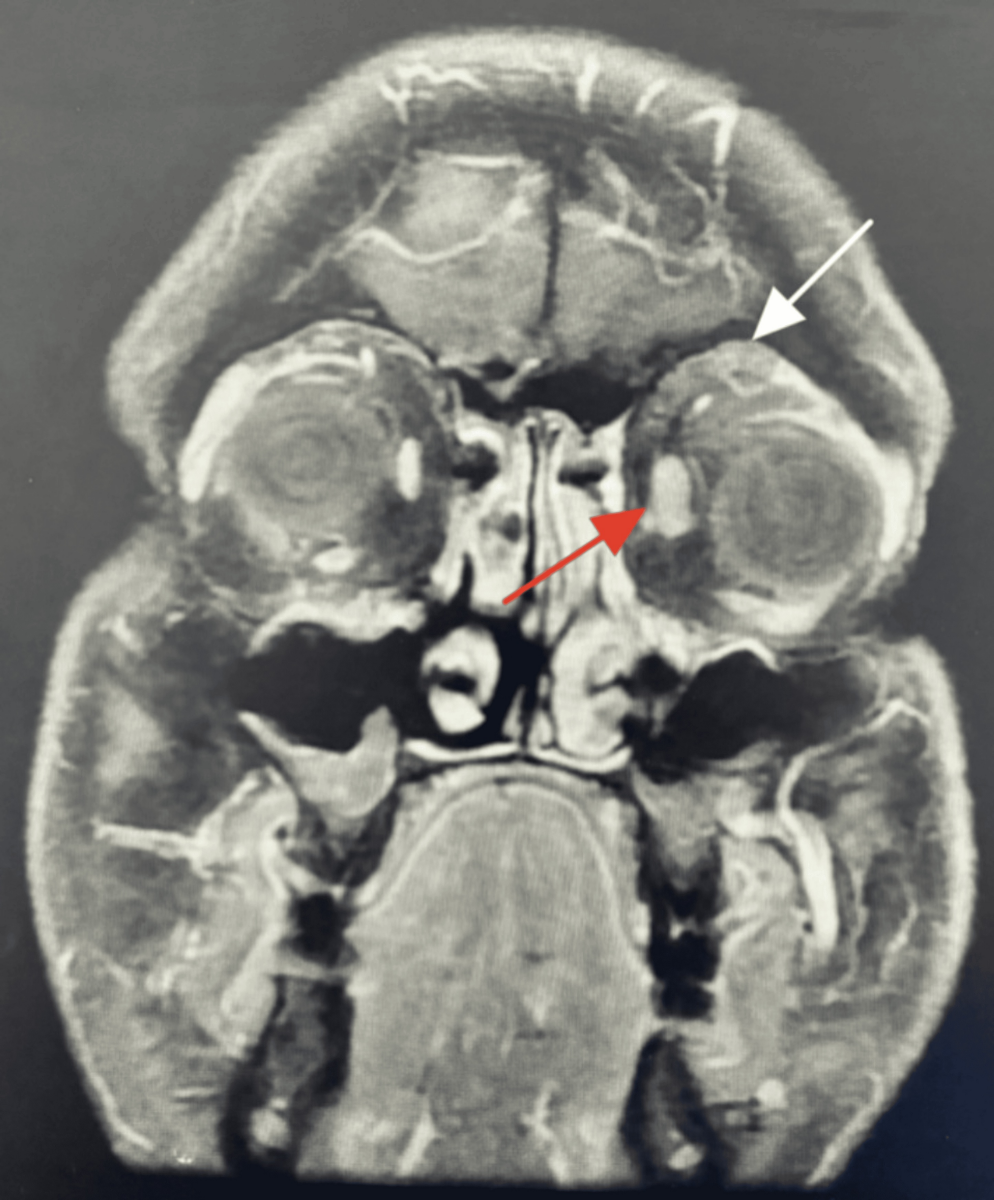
T1 coronal plane showing a mass lesion, with mixed signal intensity intermediate to low on T1-weighted image in the superior part of the left orbit and preseptal in location (white arrow). The left medial rectus muscle is mildly enlarged (red arrow)
